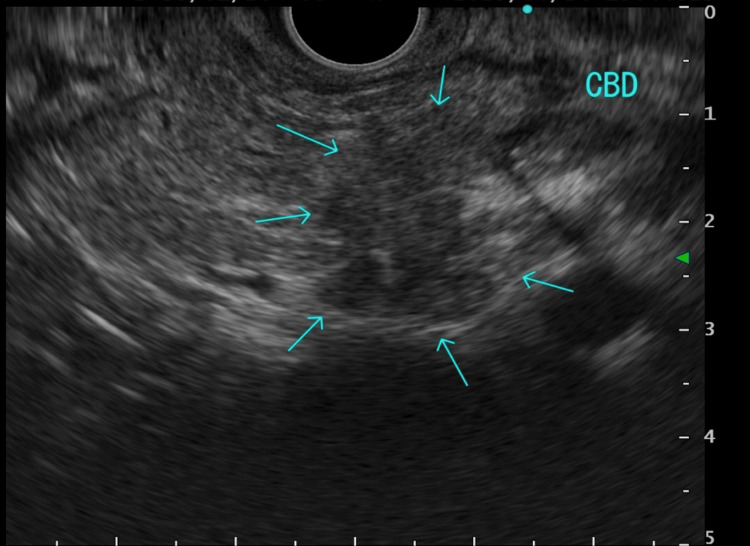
Endoscopic ultrasound of the pancreatic head (April 2023) Endoscopic ultrasound image of the pancreatic head obtained on April 24, 2023, showing a hypoechoic area with ill-defined margins and heterogeneous echotexture (arrows). No dilation of the common bile duct was observed.
